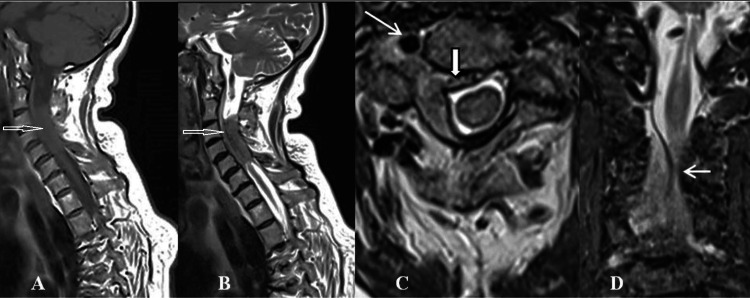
Preoperative MRI. A and B: Sagittal T1 and T2 images showing an extradural isointense tumor (thin hollow arrows). C: Axial projection C3 level. The tumor surrounds the medulla as a cuff (black and white thick arrow), penetrates the intervertebral foramen, and engulfs the right vertebral artery (white thin arrow). D: Coronal projection confirms the epidural localization of the lesion and the compression of the spinal cord (white thin arrow).
